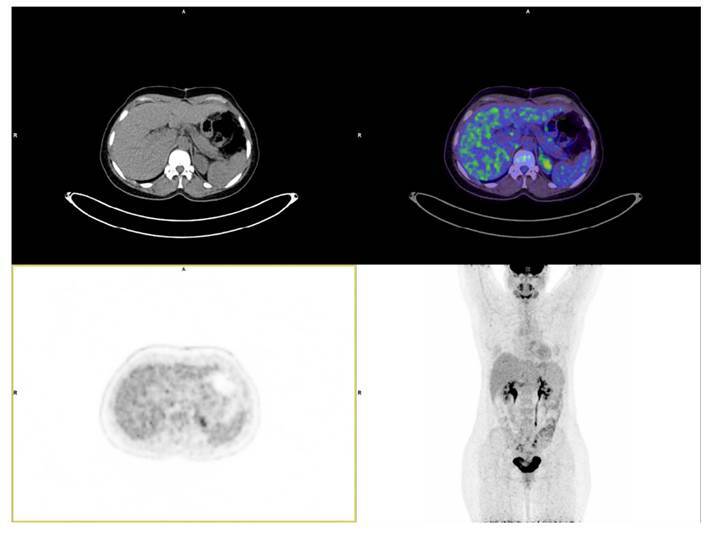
Tomografía por emisión de positrones con tomografía multicorte (PET/CT) tomada un año después de la resección tumoral. El trazador utilizado fue ^18^F-FDG. El estudio fue negativo para actividad hipermetabólica anormal.
